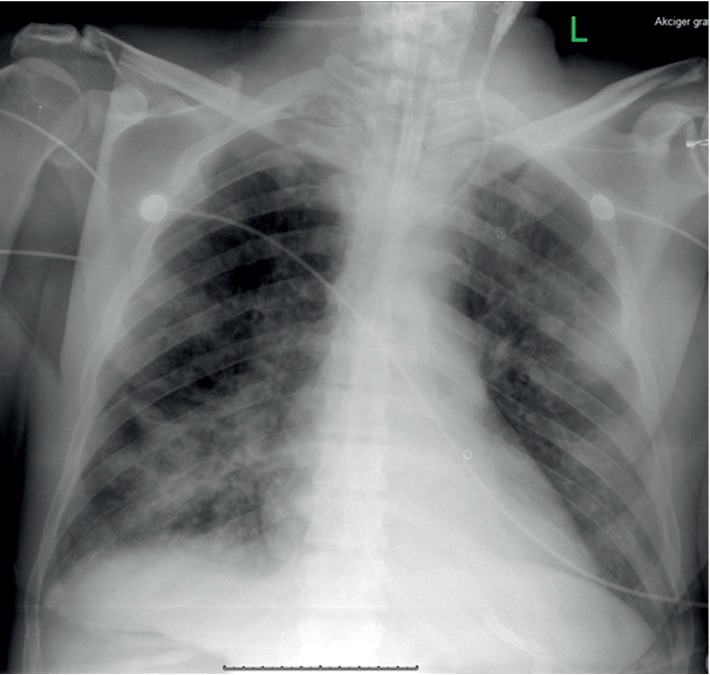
Chest roentgenogram of the patient after weaning off the ECMO.
